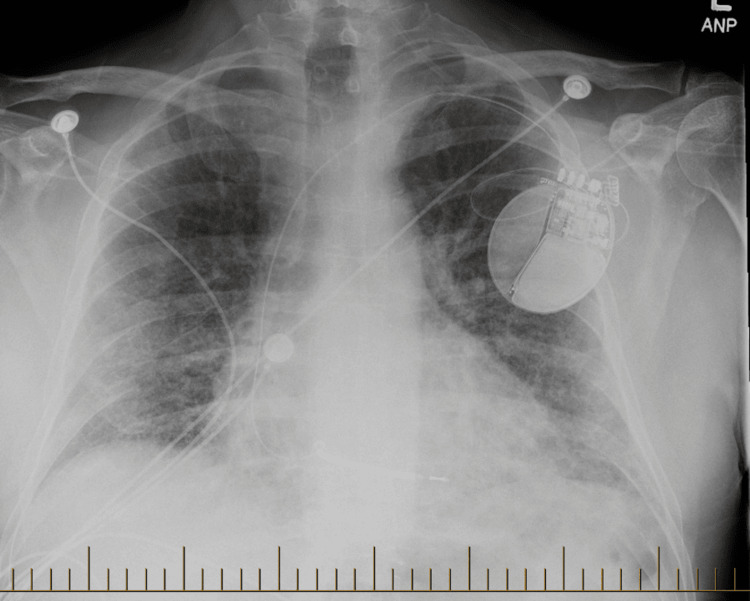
Follow-up chest X-ray obtained one month post-discharge, demonstrating bilateral improvement of pulmonary infiltrates
